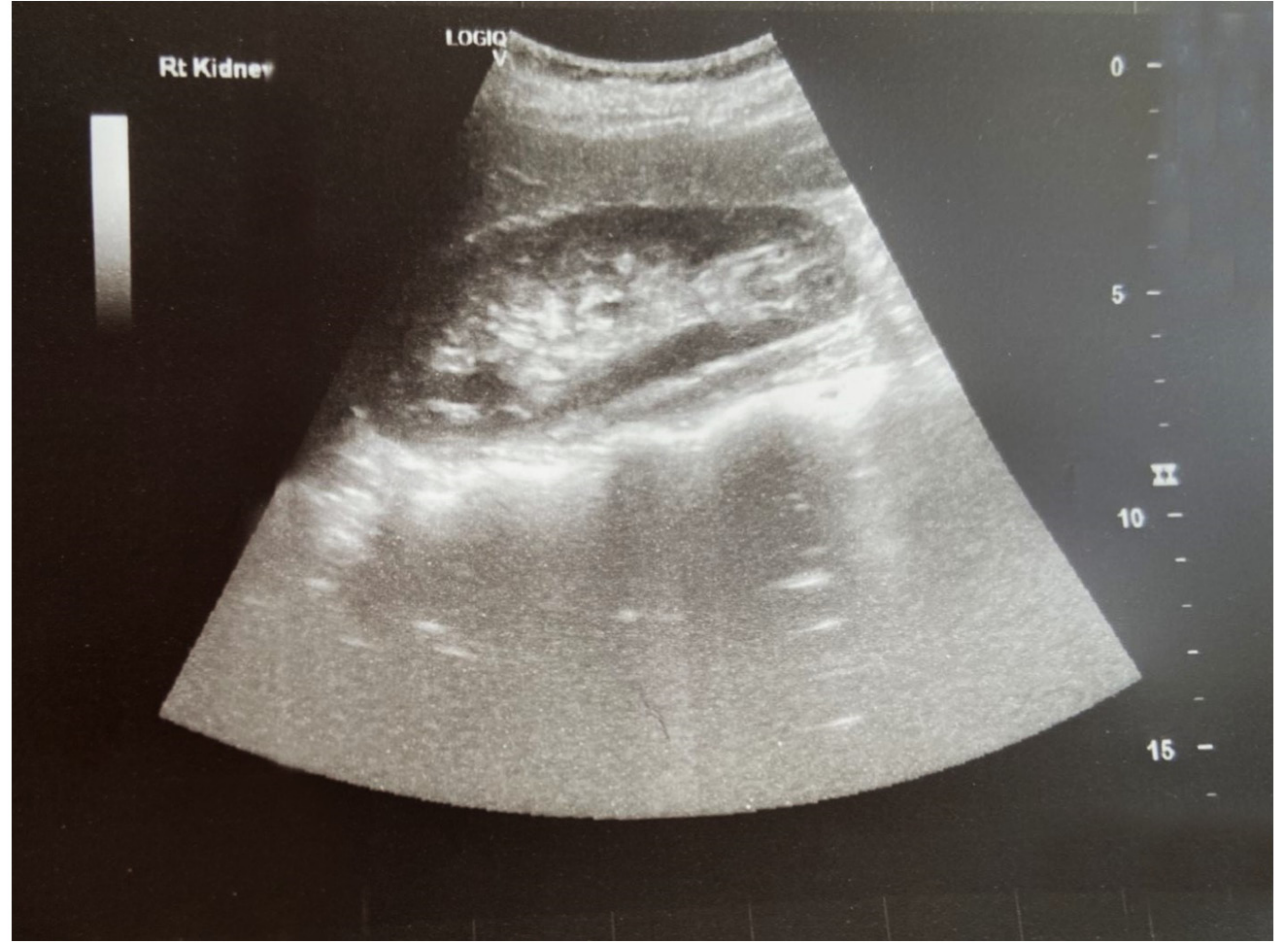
Post-operative ultrasound image of the right kidney, which confirms the absence of hydroureteronephrosis.
